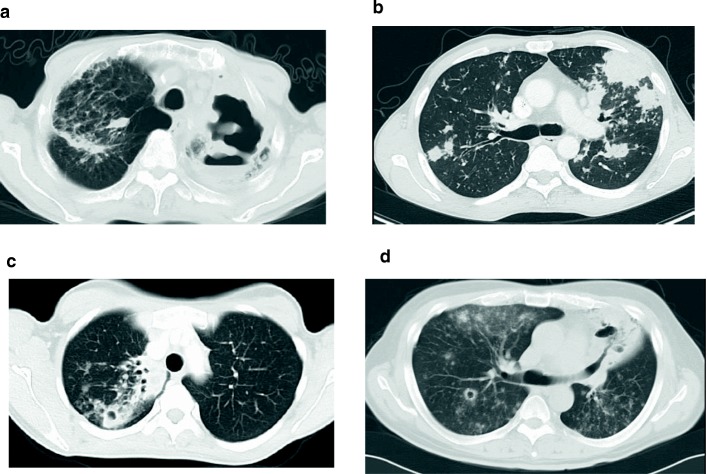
Thoracic computed tomography findings in 4 cancer patients with active *Mycobacterium tuberculosis*. a Non-small cell lung cancer patient with a thick wall cavity in the left lung with an infiltration shadow in the right upper lobe. b Colorectal cancer patient with multiple nodules in both lungs. c Breast cancer patient with a small cavity with an infiltration shadow in the right upper lobe. d Non-small cell lung cancer patient with multiple small nodules with partial patty follicular spot in the both lungs and cavity formation in the S6 segment of the right lung and lingular segment of the left lung
